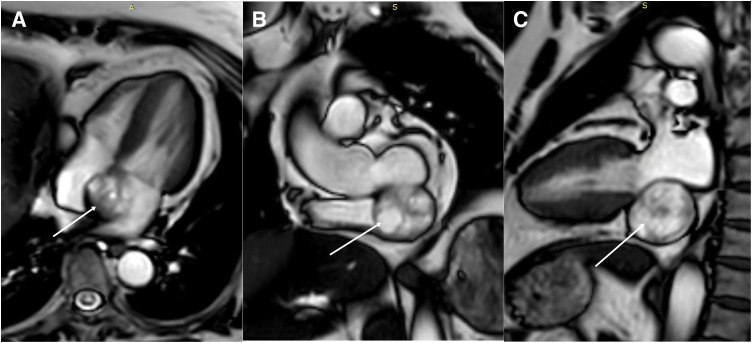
Contrast-enhanced MRI. (A) A round lesion with heterogeneous hyperintensity on T1- and T2-weighted sequences at the coronary sinus ostium in the left atrioventricular groove region; the arrow highlights the lesion. (B) Post-contrast images exhibit peripheral enhancement with internal septations; the central area remains non-enhancing, arrow indicating the tumor. (C) Well-defined margins of the lesion are clearly delineated; the arrow points to the mass.
